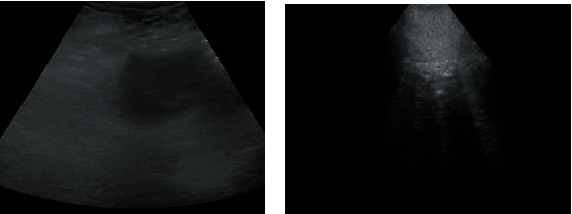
Pulmonary ultrasound. (a) Ultrasonic image 0.5 hours after extubation. (b) Ultrasonic image 20 hours after surgery.
